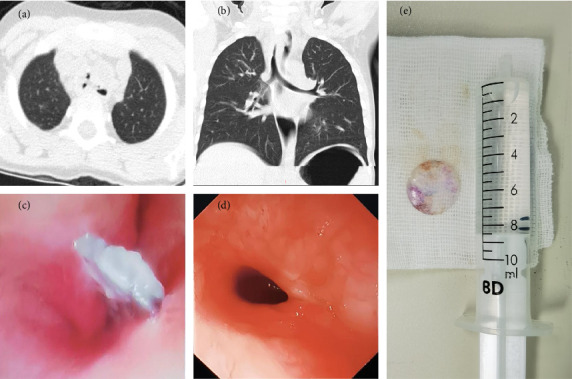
(a–b) Chest CT reveals abnormal density in the trachea and esophagus at the T1-2 level, accompanied by anterior left encapsulated gas and fluid collection, and foreign body with perforation. (c) Fiberoptic bronchoscopy reveals a foreign body at the mid-tracheal level with associated secretions. (d) Gastroscopy reveals smooth mucosa throughout the entire esophagus. (e) The foreign body is a round transparent plastic sheet, resembling a doll's eye, with a diameter of approximately 15 mm.
